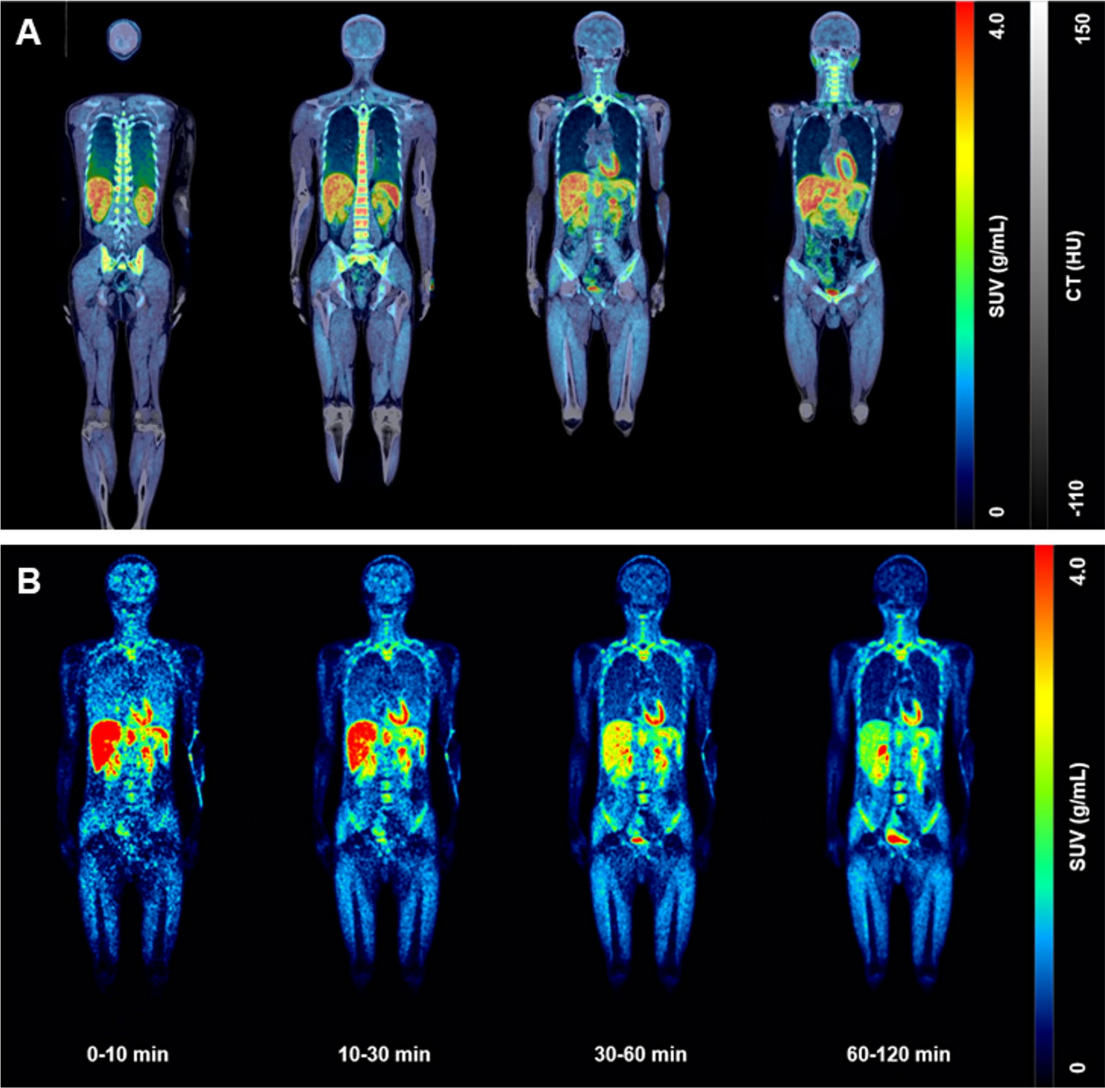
Representative decay-corrected human whole-body PET images following intravenous administration of [^18^F]LW223. (A) Average whole-body human SUV PET images (0–60 min) across multiple slices and overlayed onto CT images. (B) Whole-body human SUV PET images averaged at different time point intervals post-injection
